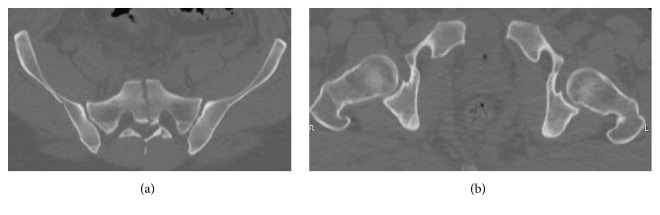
Presenting injury CT scan axial cuts ((a) and (b)).
